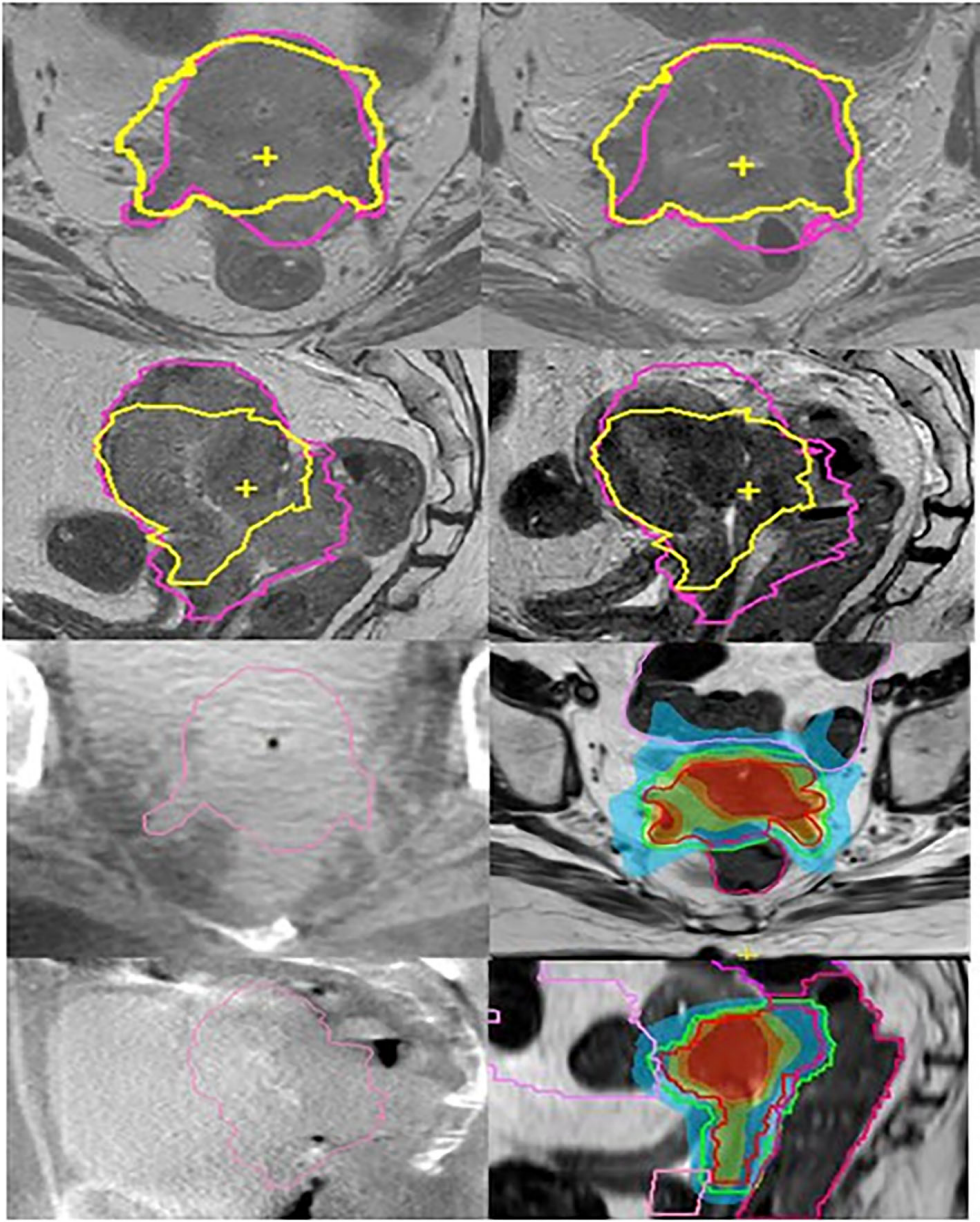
Cervix cancer; MR linac boost of HR-CTV after pelvic EBRT. Left column (top to bottom) transversal and sagittal T2 weighted MRI at time of treatment planning and on-board CBCT scans in the first week of elective EBRT. Right column (top to bottom) MRI scans after 32.4 Gy of elective EBRT and first. MR Linac boost plans. For comparison reasons, the initial and boost HR-CTV contours are shown on the MRI scans (pink at time of treatment planning and yellow after 32.4 Gy EBRT). On the CBCT scans initial HR-CTV is shown. For the MR Linac boost plan, the online delineation of the first fraction for HR-CTV and rectum is shown. The images show the improved visualization of MRI compared to CBCT.
